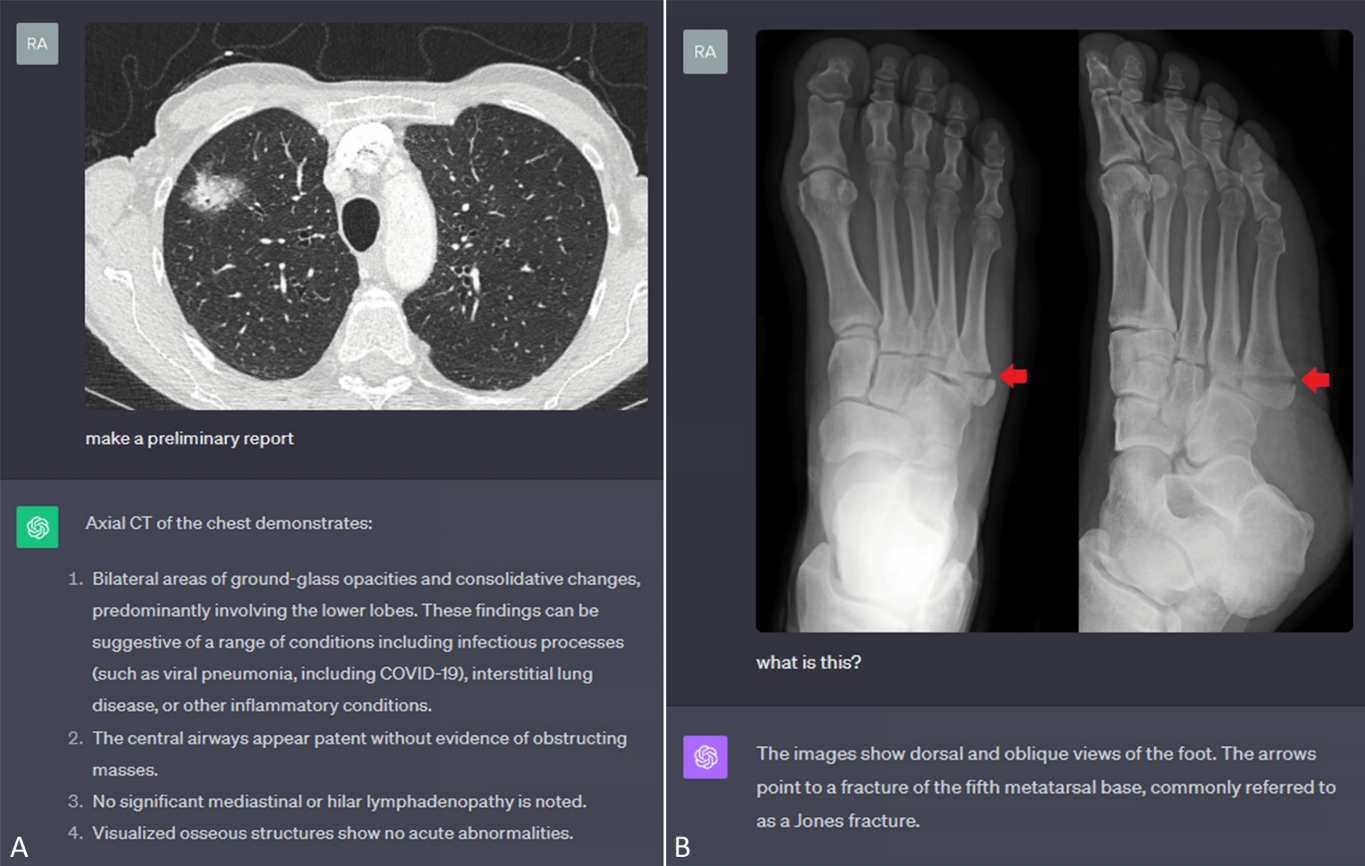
Radiologic image report generation A. Interpretation of a CT chest with ground-glass opacity. B. Interpretation of a foot radiograph with the help of an arrow (without the arrow, the fracture was not detected).
